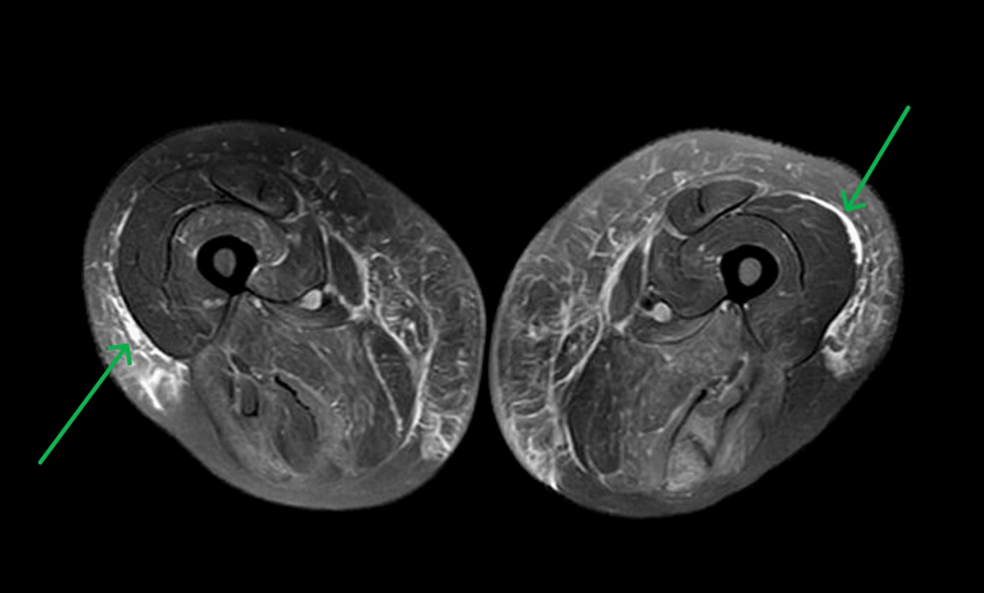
Axial T2-weighted MRI of the proximal lower extremities demonstrating hyperintensities involving all muscle compartments indicative of non-specific edema.
